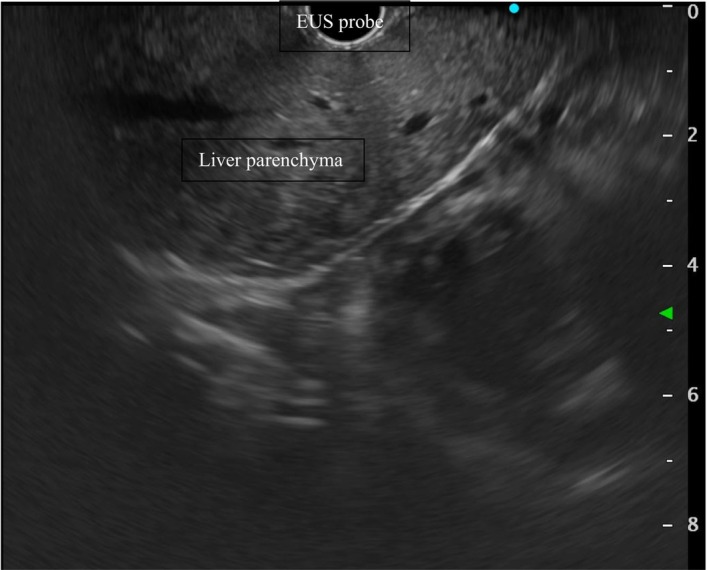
Endoscopic ultrasound image of the hepatic left lobe in liver cirrhosis. The image is obtained from the cardial region.
